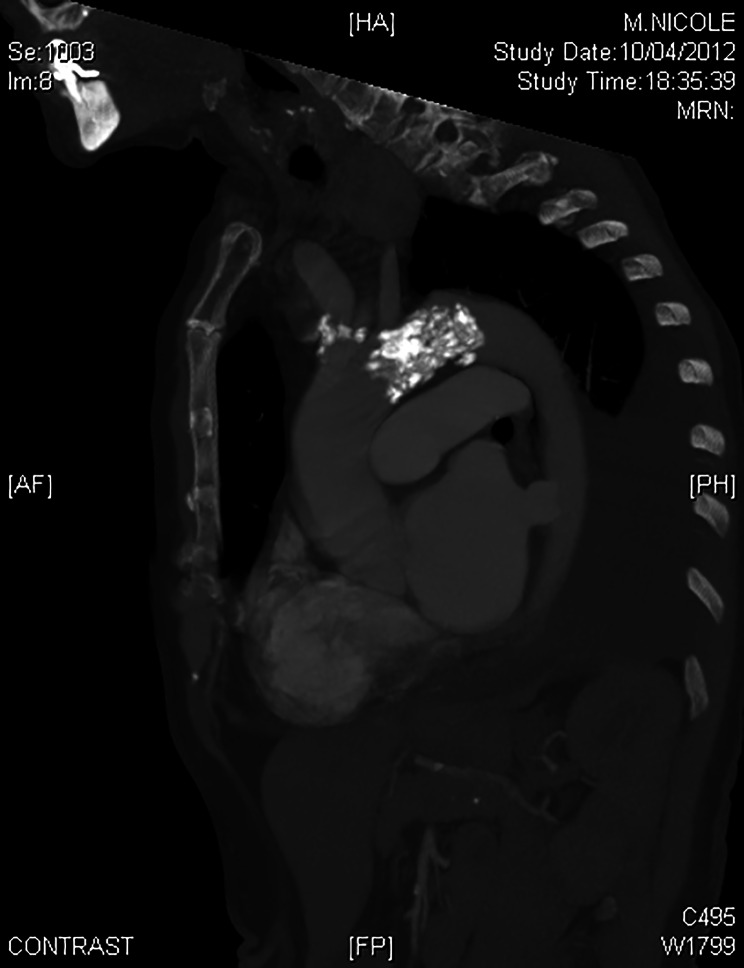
CT scan (sagittal cut) showing the calcified plaque rising up to the aortic arch in front of the left subclavian artery emergence
